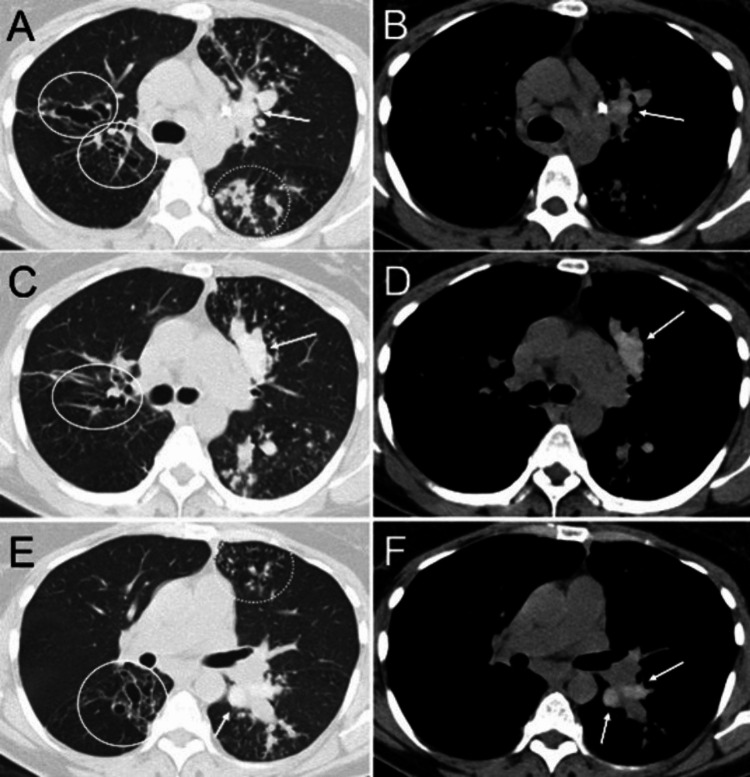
Allergic bronchopulmonary aspergillosis in a patient with asthma, environmental allergies, chronic persistent cough, and positive skin prick test for Aspergillus. Axial CT images using lung window settings (A, C, E) and mediastinal window settings (B, D, F) demonstrate central and upper lobe predominant bronchiectasis (solid circles), high-attenuation mucus plugs (arrows), and centrilobular nodules with a tree-in-bud pattern (dashed circles).
